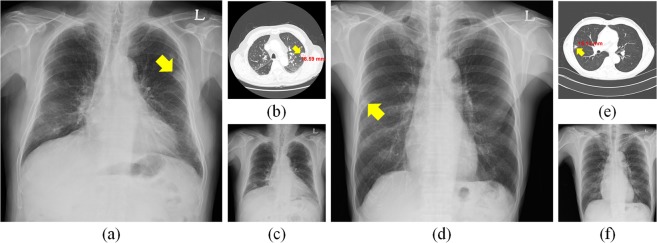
Initial and follow-up (F/U) CXRs and CT images with a nodule. (a) Initial CXR in a 65-year-old male patient with 18.59 cm metastatic renal cell carcinoma (arrowhead) in the left upper lobe and (b) CT examination of patient (a). (c) F/U CXR of (a). (d) Initial CXR of an 81-year-old male patient with 15.13 cm metastatic adenocarcinoma (arrowhead) in the right middle lobe and (e) CT examination corresponding to (d). (f) F/U CXR of (d).
